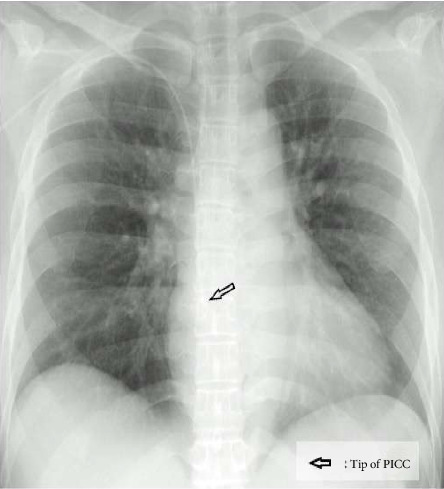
A confirmatory chest radiograph taken after insertion of the peripherally inserted central venous catheter (PICC).
